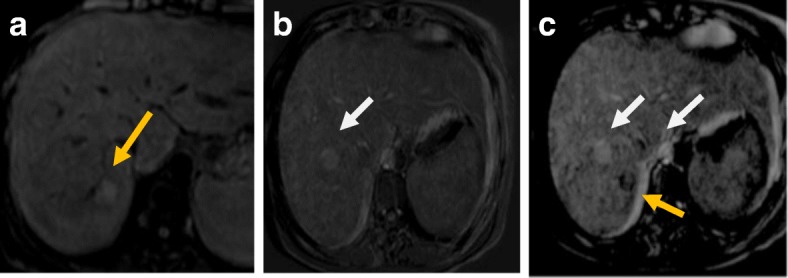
MRI after RF. a Pre-contrast T1-WI fat-suppressed image shows the ablated HFL at subsegment VII (yellow arrow) with its bright signal due to coagulative necrosis. b Subtracted arterial phase at a higher level shows slice mis-registration between the pre-contrast and post-contrast image and mirror image of the aorta (white arrow). c Subtracted arterial phase at the HFL shows slice mis-registration between the pre-contrast and post-contrast image and mirror image of the aorta (white arrow) with eccentric faint enhancement at the lesion (not consistent with the residual viable tumor). Final diagnosis is LR-TR nonevaluable due to image degradation
